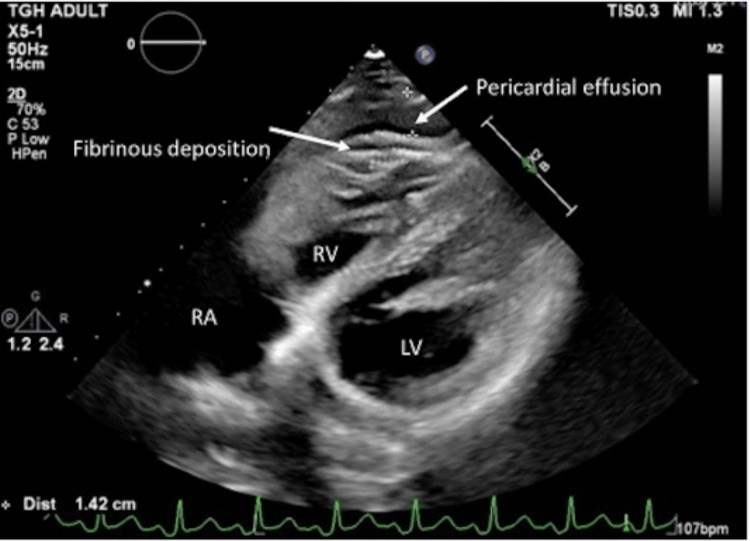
Transthoracic echocardiogram demonstrating small to moderate anterior pericardial effusion with associated fibrinous deposition
